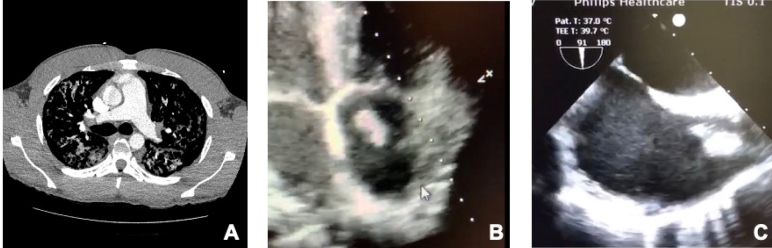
CTPA with filling defects in the pulmonary arteries bilaterally (A), transthoracic (B), and transesophageal (C) echocardiography showing thrombus in the right atrium. CTPA: computed tomography pulmonary angiogram.
